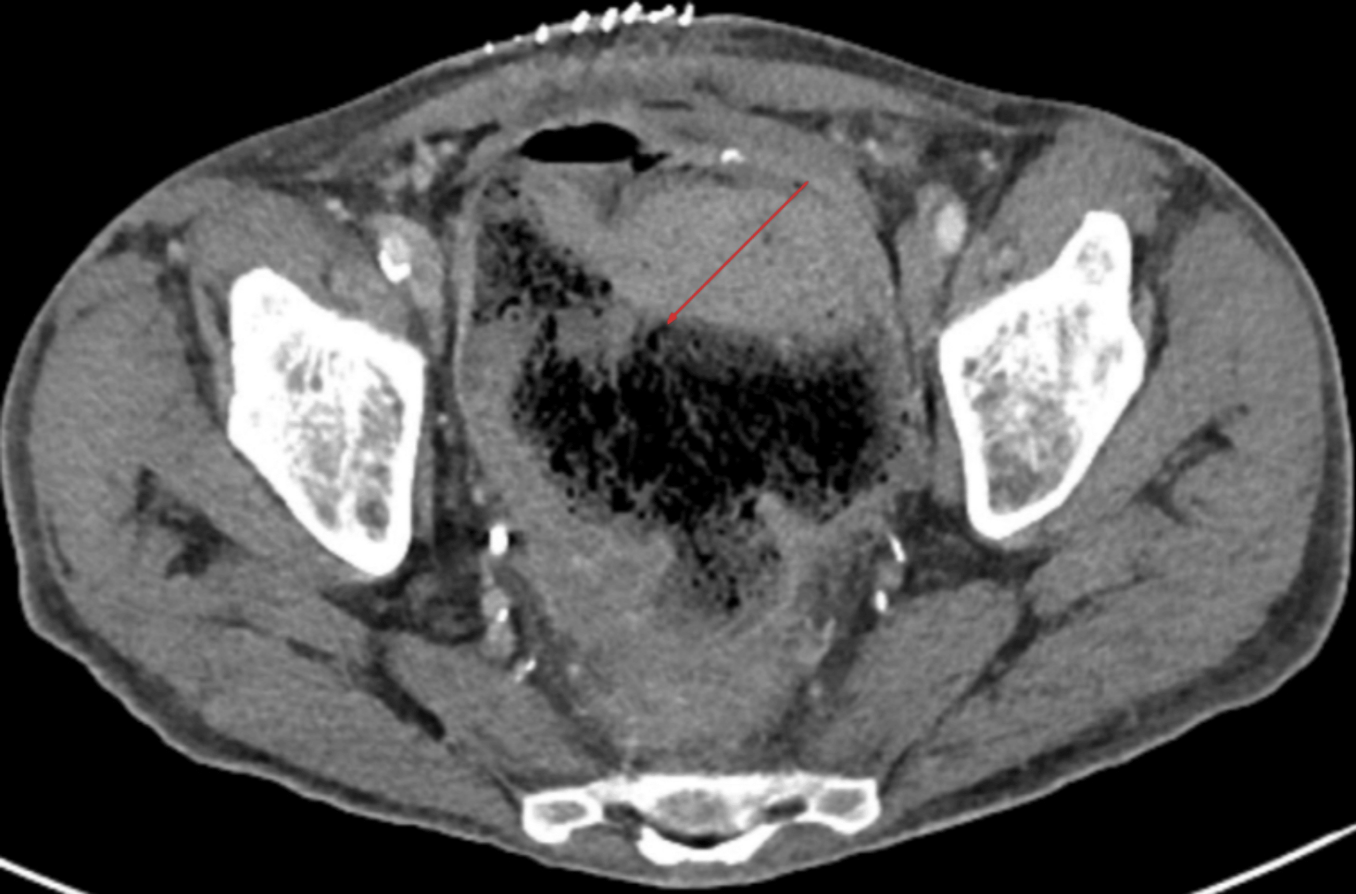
CT abdomen/pelvis in axial view demonstrating a large collection with an air-fluid level and feculent material (red arrow) in the right lower abdomen and pelvis CT: Computed tomography
